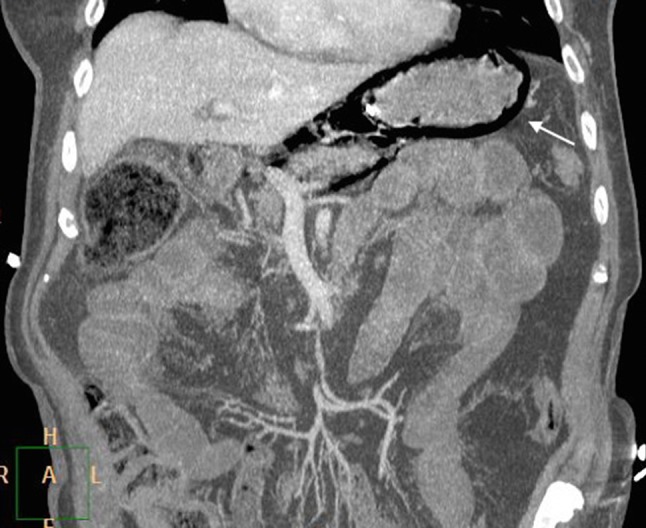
Contrast-enhanced CT, MPR reformats. Large amounts of intramural gas in the stomach (arrow) with no evidence of free peritoneal air.
